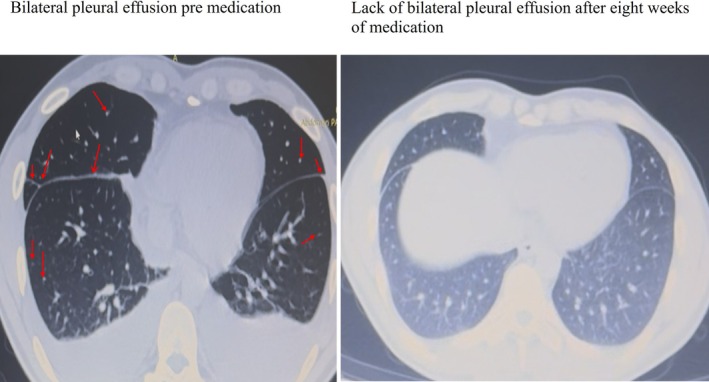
Baseline chest CT demonstrating bilateral pleural effusion and symmetrical mediastinal and hilar lymphadenopathy. Red arrows indicate multiple small nodules with a perilymphatic distribution.
